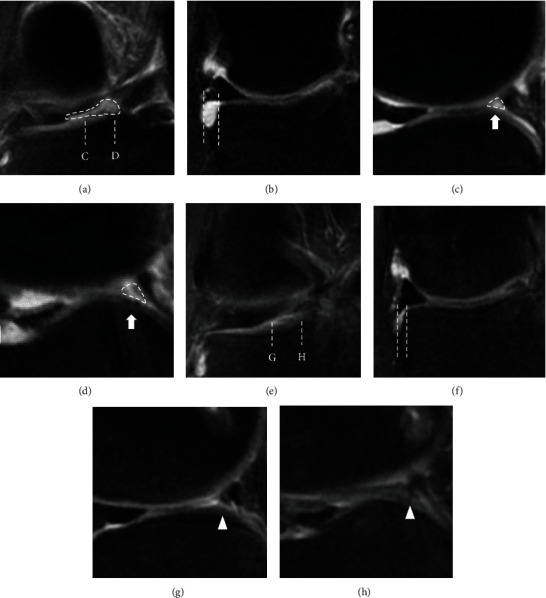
Comparison of the (a–d) preoperative and (e–h) postoperative MRI findings. (a) Preoperative MRI showed a linear defect in the coronal plane (dotted area). (b) The length of the lateral meniscus extrusion (LME) was 3.1 mm (length between dashed lines). (c, d) The reference lines of the sagittal plane are shown as dotted lines in (a) (C and D, respectively). Ghost sign in two consecutive sagittal planes with a 10 mm interval (dashed area). (e) One year after the operation, the preoperative gap was filled with tissue continuous to the tibial attachment of the LMPR in the coronal plane. (f) The length of LME was 1.6 mm (1 year postoperatively). (g, h) The reference lines of the sagittal plane are shown as dotted lines in (e) (G and H, respectively). The disappearing ghost sign in two consecutive sagittal planes with a 10 mm interval (arrowhead).
